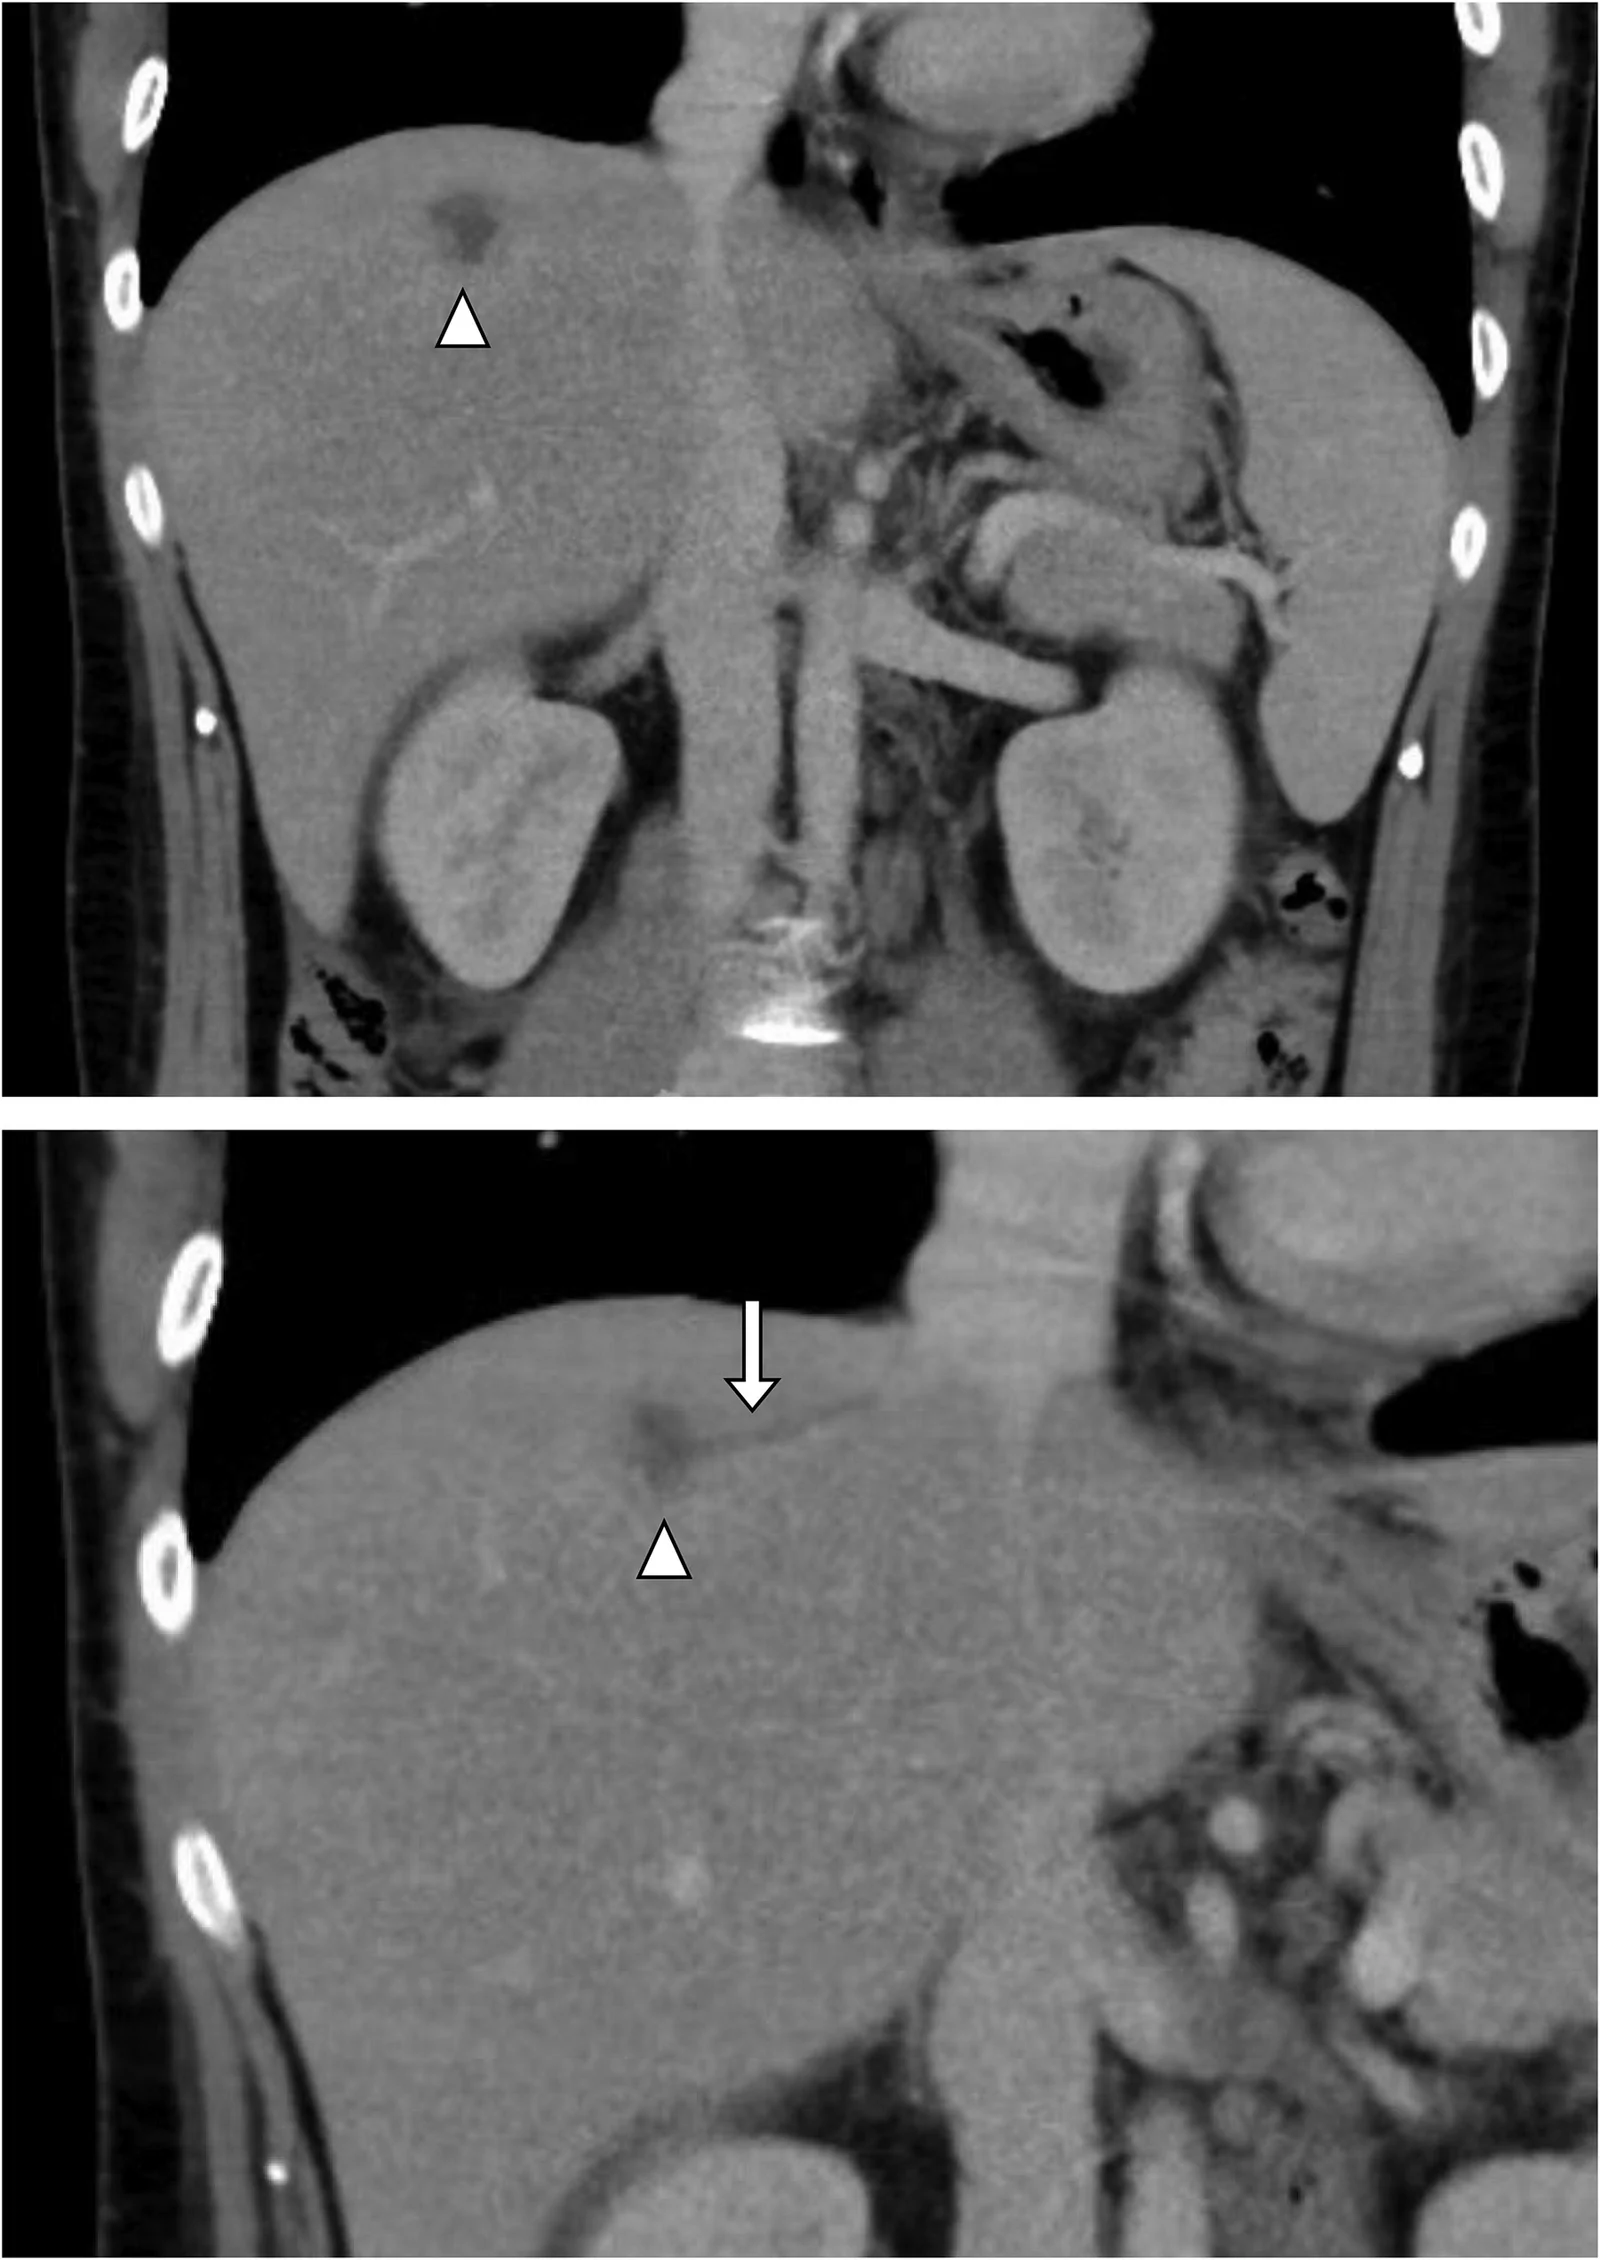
Coronal abdominal CT demonstrating the hepatic S8 dome abscess (arrowhead) with adjacent hepatic vein thrombophlebitis (arrow), identified by wall thickening and enhancement of the hepatic vein with internal low density, findings supporting infected (septic) thrombus rather than bland thrombosis, which would instead show an extensive thrombus burden, venous obstruction, or a hepatic perfusion abnormality, none of which were present on this study.
